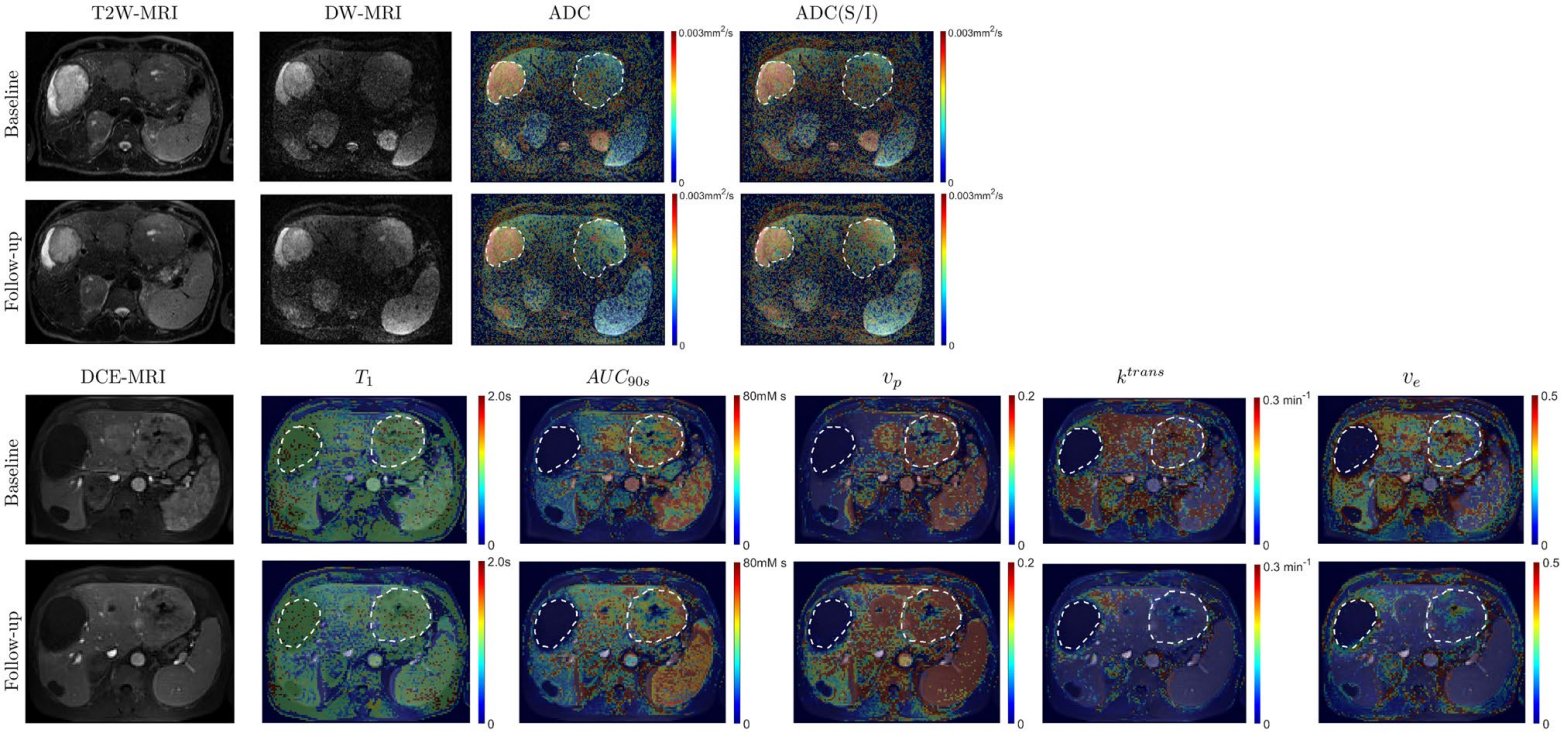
Quantitative parameters maps before and after treatment of a subject with metastatic hemangiopericytoma. There was significant replacement of healthy liver by tumor in this subject. T2-weighted fat-suppressed, DW-MRI ($$b=0\,\text{s/mm}^{2}$$), and DCE-MRI (2^nd^ time point post-injection of contrast) images are shown for anatomic reference, along with maps of pre-contrast T1, ADC, ADC(S/I), $${AUC}_{90s}$$, $${v}_{p}$$, $${k}^{trans}$$ and $${v}_{e}$$.
